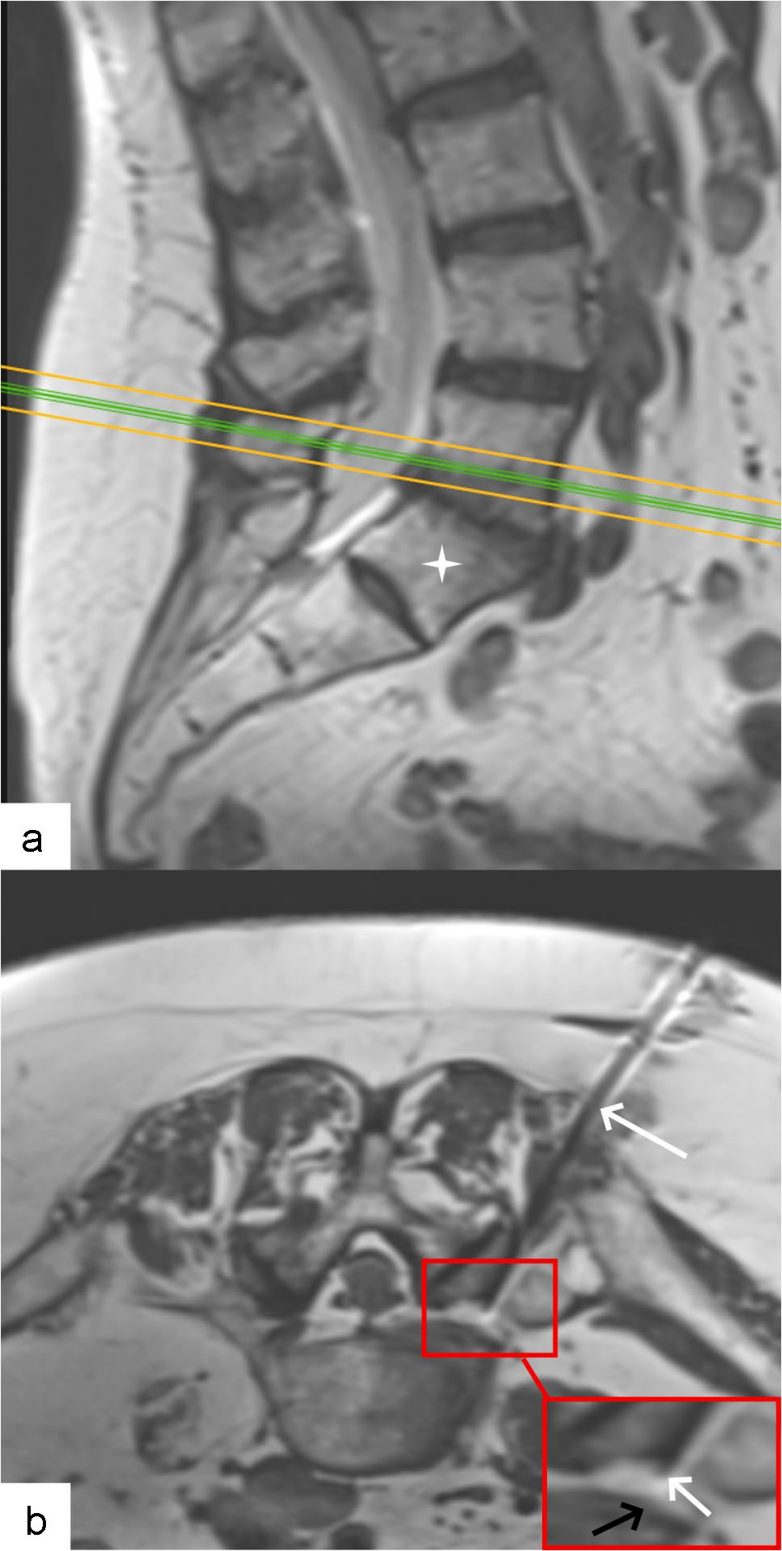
Non-contrast MRI scan obtained in nerve root infiltration in 70-year-old woman. a Sagittal T2 turbo spin echo-weighted image showing oblique cranioventral access plane (green line) chosen to access L5 nerve within L5-S1 neural foramen. Note transitional lumbo-sacral vertebra. b Axial oblique proton density-weighted image showing 20G needle (white arrow) with tip (arrowhead, inset) arriving behind left L5 nerve (black arrow, inset)
